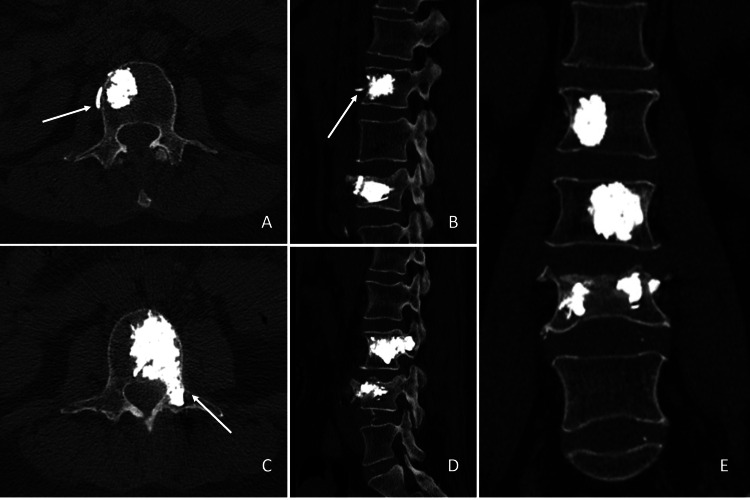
Postoperative computed tomography of the lumbar spine A and C, axial images; B and D, sagittal images; E, coronal image. On the first postoperative day, computed tomography of the lumbar spine confirmed the correct positioning of the cementing material in both vertebral bodies and the left L3 peduncle. A. Axial image of L2. B. Sagittal image of the lumbar spine, paramedian right. The anatomical vessel distribution of the hyperdense PMMA allows appreciation of the obliteration of the vascular afferents (white arrow). C. Axial image of L3. Hardening of the left peduncle was achieved (white arrow). D. Sagittal image of the lumbar spine, paramedian left. E. Coronal image of the lumbar spine.
